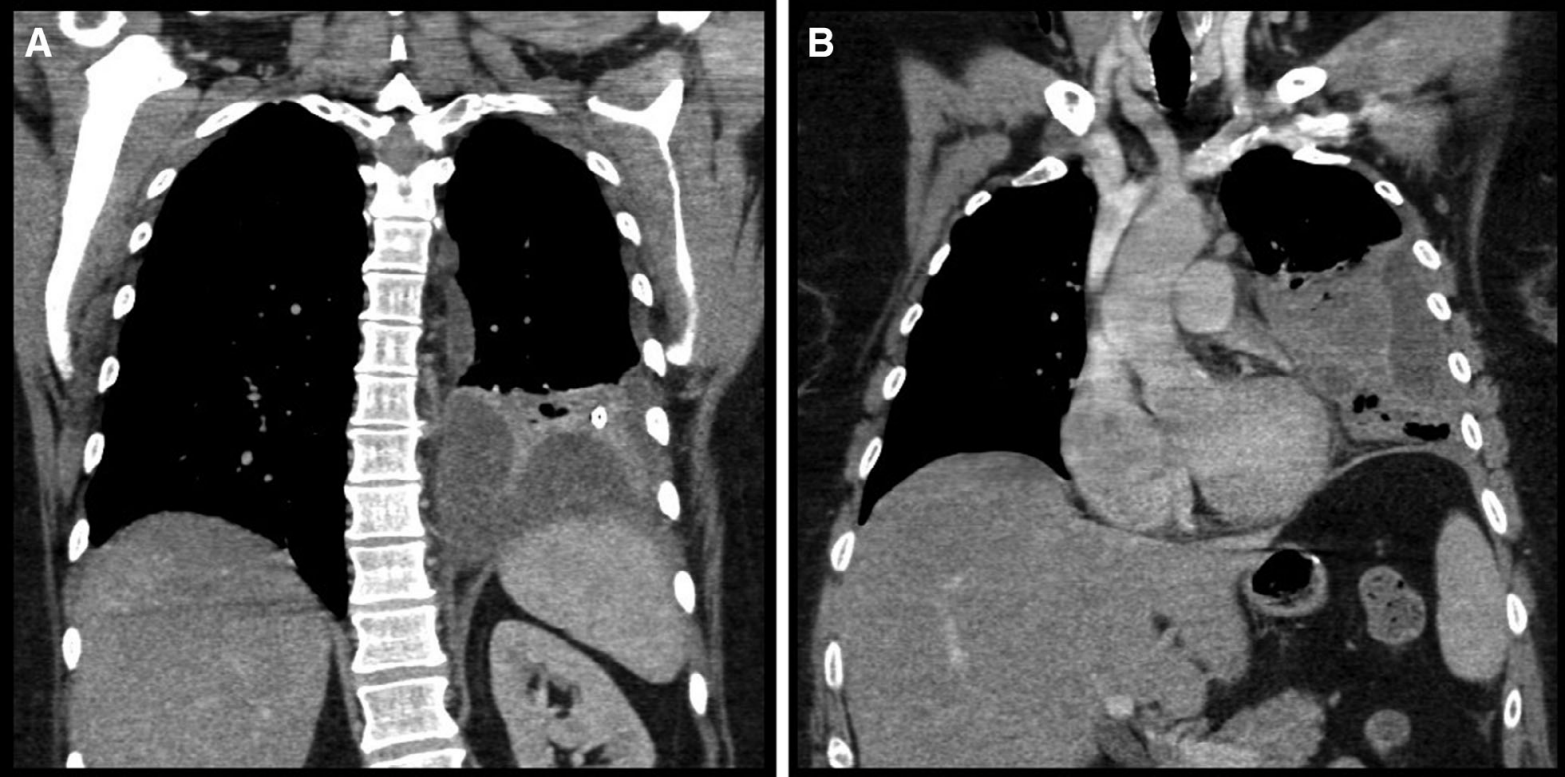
Computed tomography (CT) images at initial presentation demonstrating separate (A) basal and (B) lateral pleural fluid collections.
